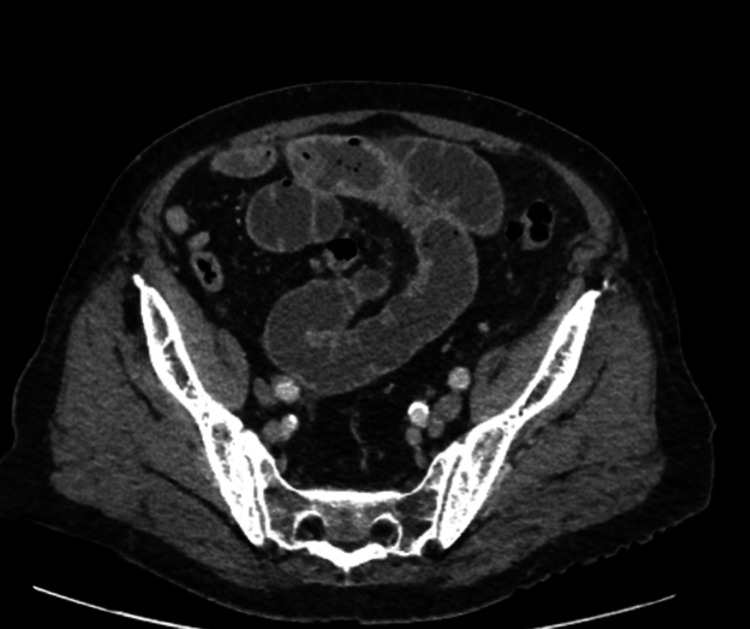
Computed tomography scan on admission showing the distended proximal small bowel and collapsed colon.
